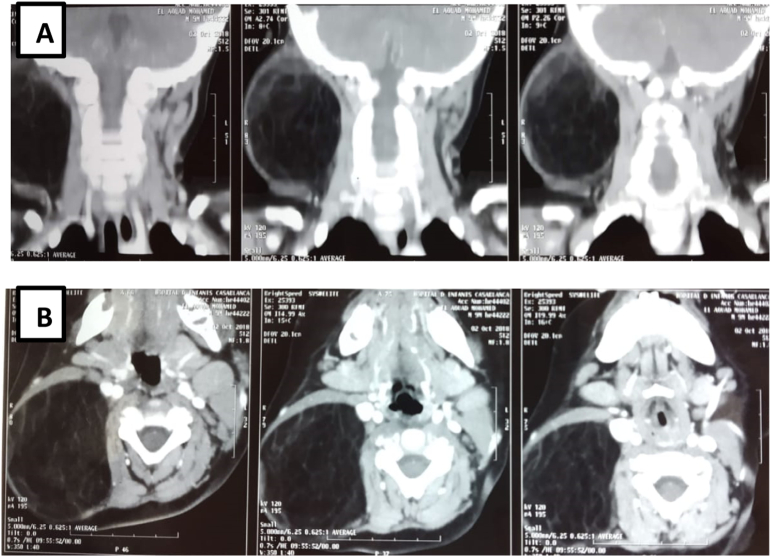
Neck CT scan, A/ coronal, B/ axial section: a well limited right latero cervical mass of a greasy density (61 × 50 × 65 mm) crossed by multiple septa.
